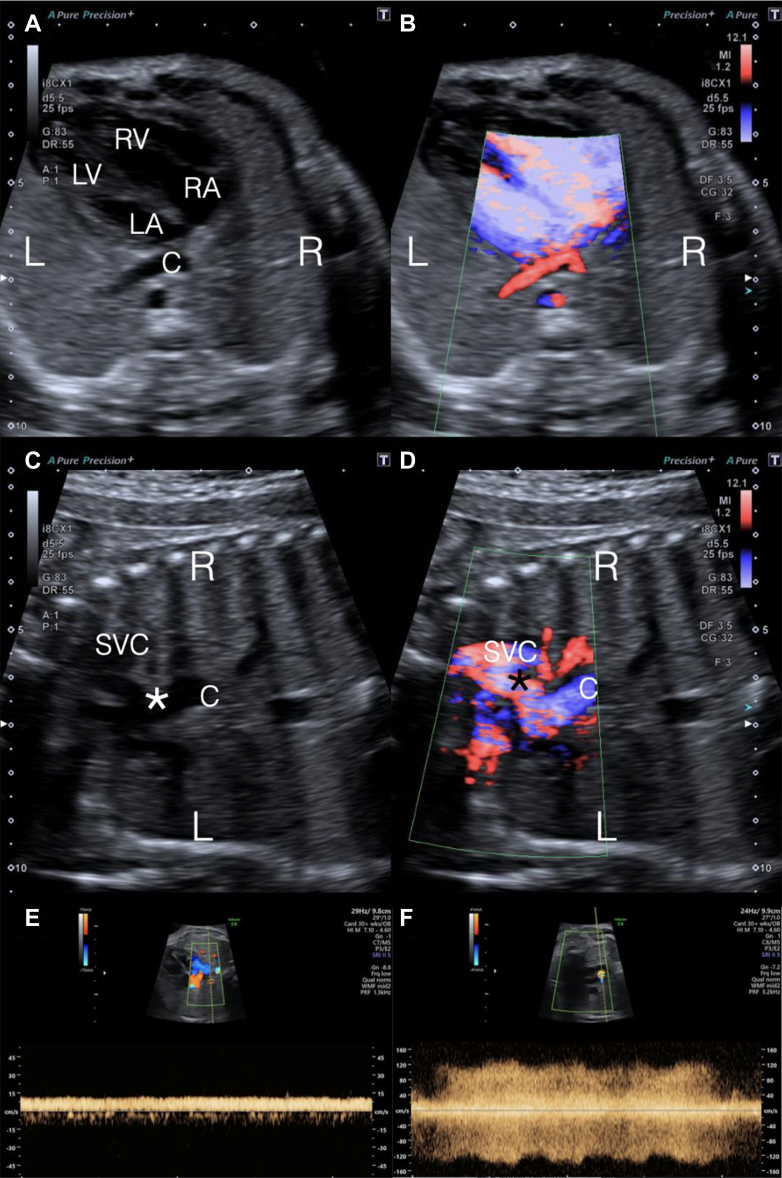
Fetal Echocardiography at 32 Weeks of Gestation (Case 1) (A) Four-chamber view. (B) Four-chamber view with power color Doppler. (C) Coronal view (fetal head to the left). (D) Coronal view with power color Doppler. There is a confluence (C) behind the left atrium (LA) with no direct connection of the pulmonary veins to the cardiac mass. The confluence drains via an ascending vein (asterisk) to the junction of the innominate vein and superior vena cava (SVC). Doppler assessment showed a flat, nonphasic signal in the pulmonary veins (E) with an area over turbulence in the ascending vein (F). L = left; LV = left ventricle; R = right; RA = right atrium; RV = right ventricle.
